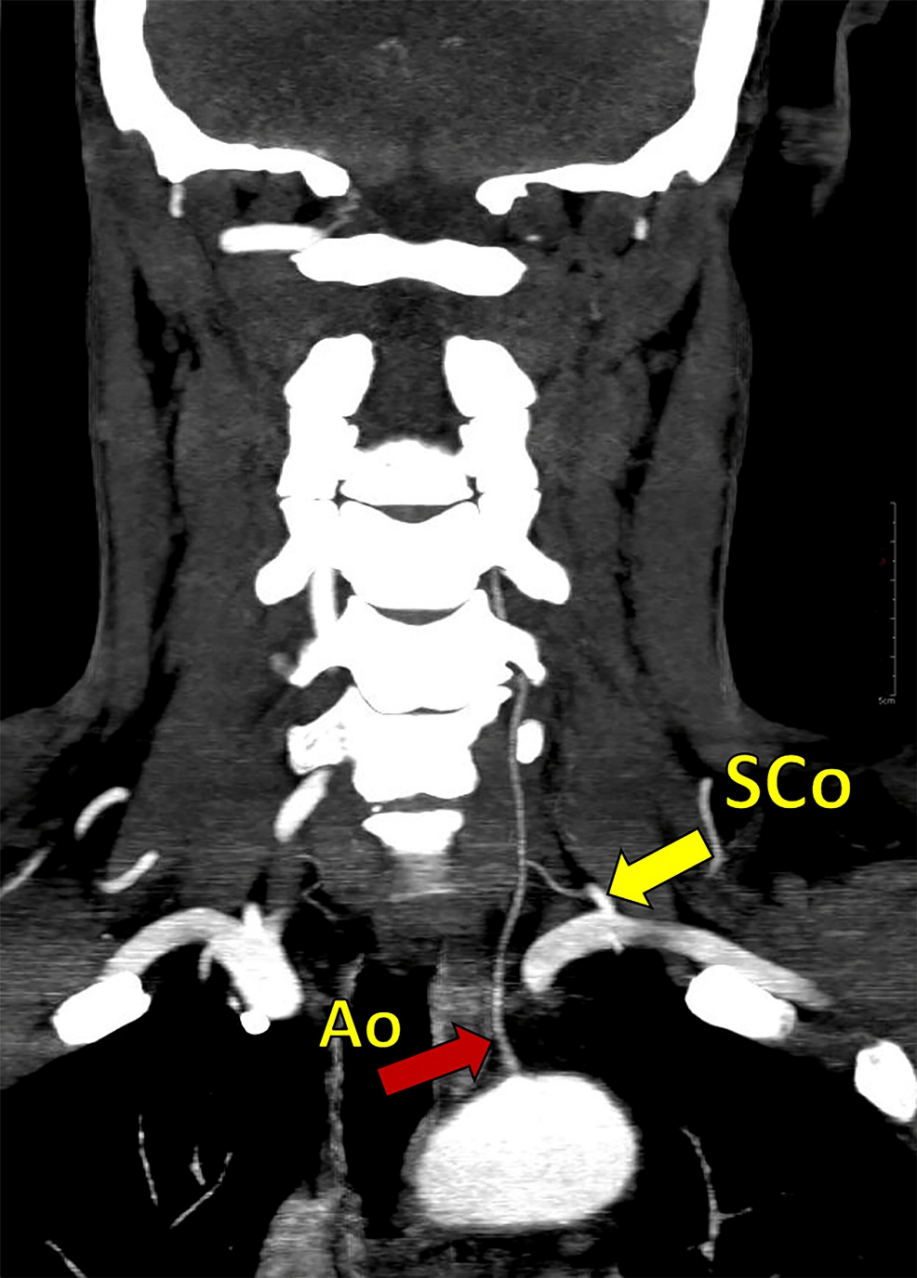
CT angiographic image showing dual origin (aortic: red arrow, Ao; subclavian origin: yellow arrow, SCo) of the vertebral artery on the left side. Note: Both origins were hypoplastic.
